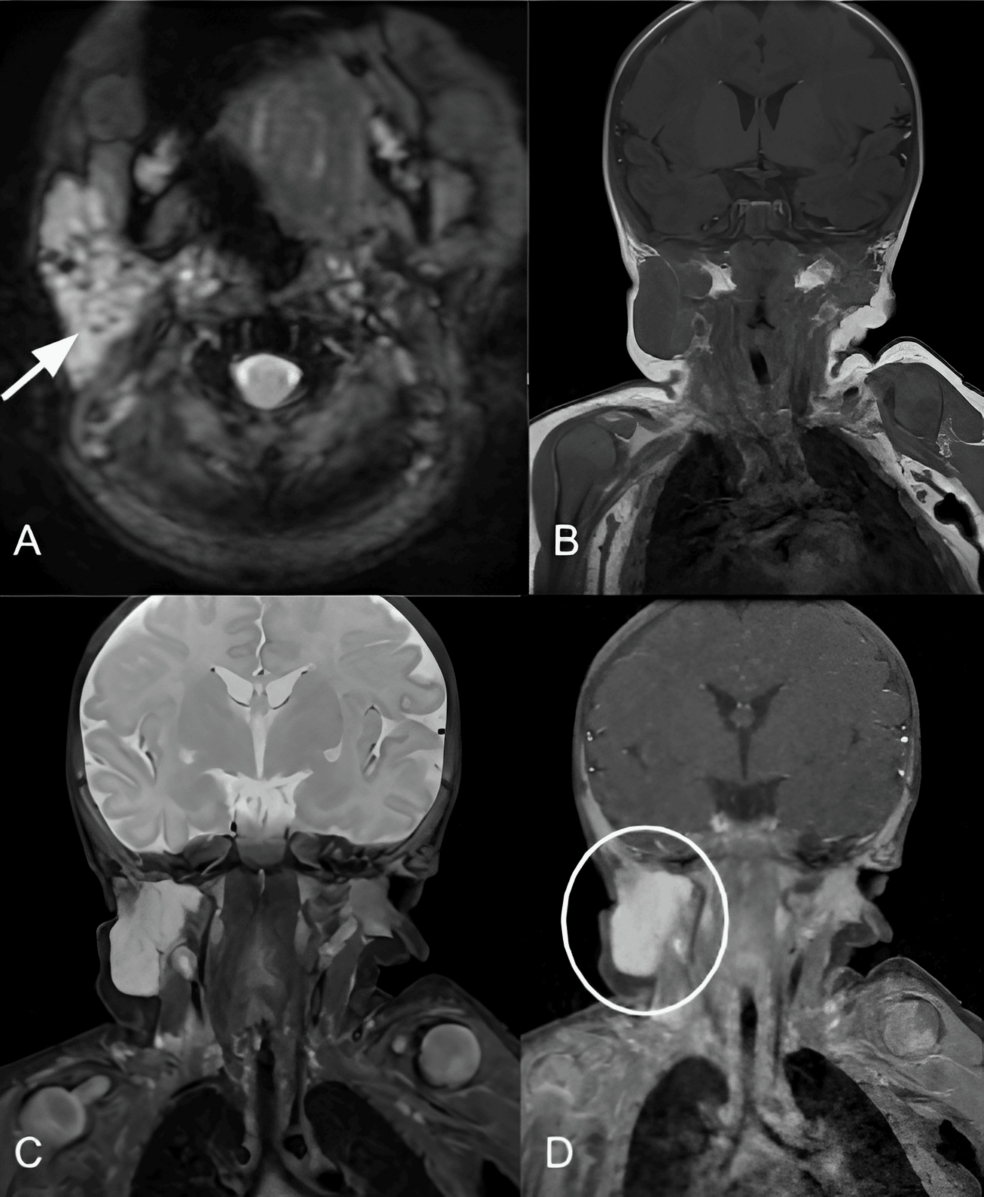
MRI of the Brain and Face. Axial T2-weighted (A), coronal T1- (B), T2-weighted (C) and gadolinium-enhanced image (D) showed a lobulated mass centered in the right parotid gland, hypointense in T1, hyperintense in T2 with homogeneously strong and early enhancement. Notice the prominent flow voids within the gland which correspond to vessels (arrow in A). No infiltration of the surrounding structures.
